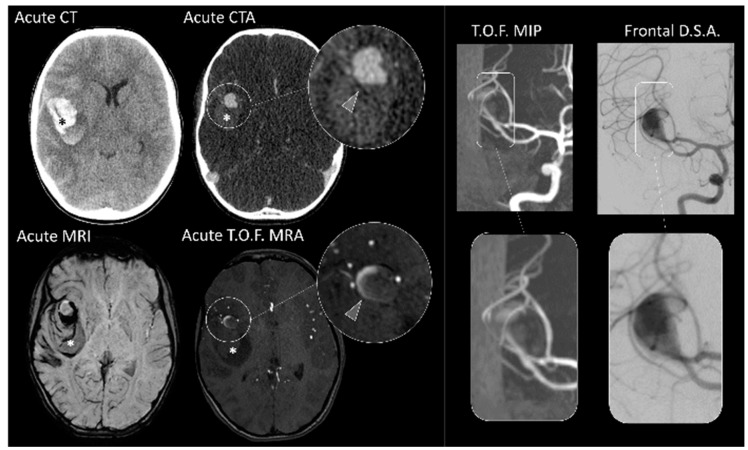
Vascular imaging in pediatric ICH. Admission non-contrast CT with intracerebral hemorrhage (asterisk). Corresponding CTA shows evidence of large aneurysm of the right MCA. In the MRI (bottom row and right panel)) of the same patient, TOF-angiography does not clearly show the aneurysm. The DSA clearly visualizes aneurysm morphology.
